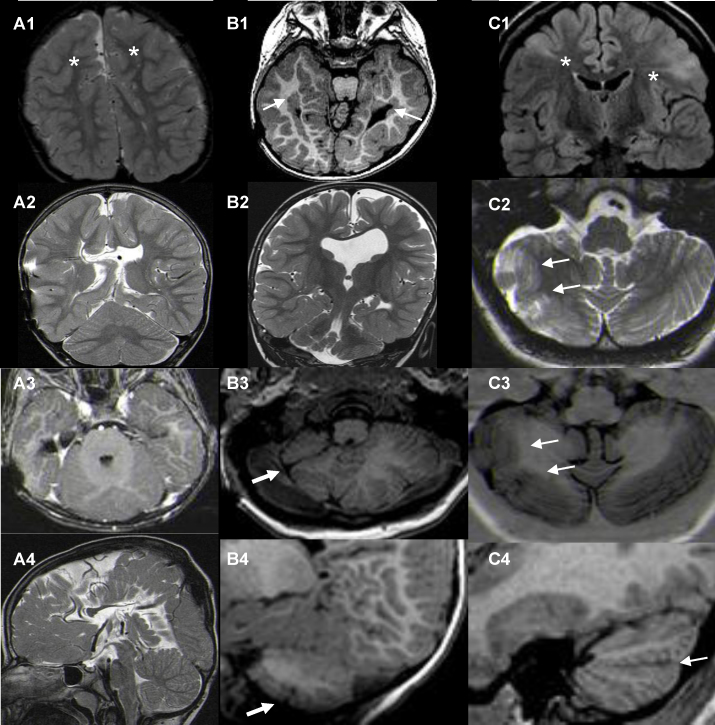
Cerebellar dysplasia. First column (A1–4) – rhombencephalosynapsis. (A1) Axial T2-weighted image with bilateral frontal polymicrogyria (asterisk); (A2) T2-weighted coronal and (A3) T1-weighted axial images show dorsally fused cerebellar hemispheres and superior cerebellar peduncles; (A4) T2-weighted sagittal image. Second column (B1–4) – cerebellar polymicrogyria with cleft. (B1) Axial T1-weighted image with bilateral periventricular nodular heterotopia along temporal horns of lateral ventricles (thin white arrows); (B2) coronal T2-weighted, (B3) axial T1-weighted and (B4) sagittal T1-weighted images show irregular structure of cerebellum in three different planes. (B2) Hypoplastic/partially infolded hippocampi. Cleft with overlying polymicrogyria (thick white arrows in B3 and B4) of the right cerebellar hemisphere (right hemisphere is smaller compared to the left one). Third column (C1–4) – cerebellar polymicrogyria with cleft. (C1) subcortical tubers on coronal FLAIR image (white asterisk); (C2) axial T2-weighted and (C3) T1-weighted images show two sites of cerebellar polymicrogyria with cleft on the right (white arrows). (C4) sagittal T1-weighted image corresponding to the section on axial T2-weighted (C2) and T1-weighted (C3) images with a deep fissure and polymicrogyric cerebellar cortex (white arrow).
